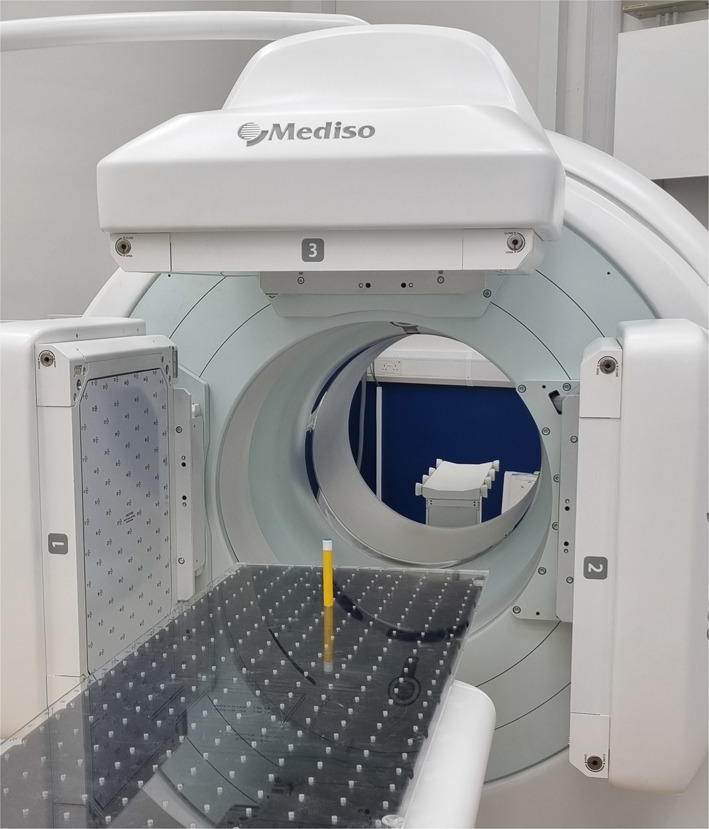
A photograph showing one of the calibration ampoules in a reproducible position on a custom-made perspex board beneath a stationary SPECT head of the Mediso AnyScan SPECT system. The same position was used for each source
